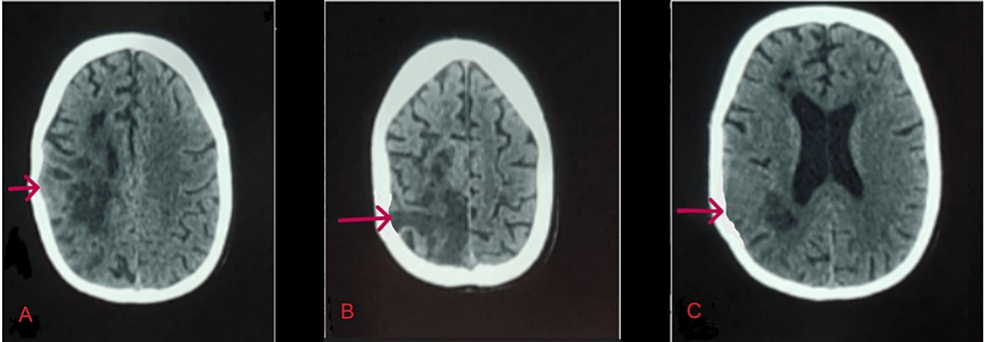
Non-contrast computed tomography of the patient. (A, B) NCCT brain showing mild hypo density with loss of gray-white matter differentiation in the right posterior frontoparietal region. (C) Chronic ischemic foci in right frontal and parietal periventricular white matter. NCCT: non-contrast computed tomography
